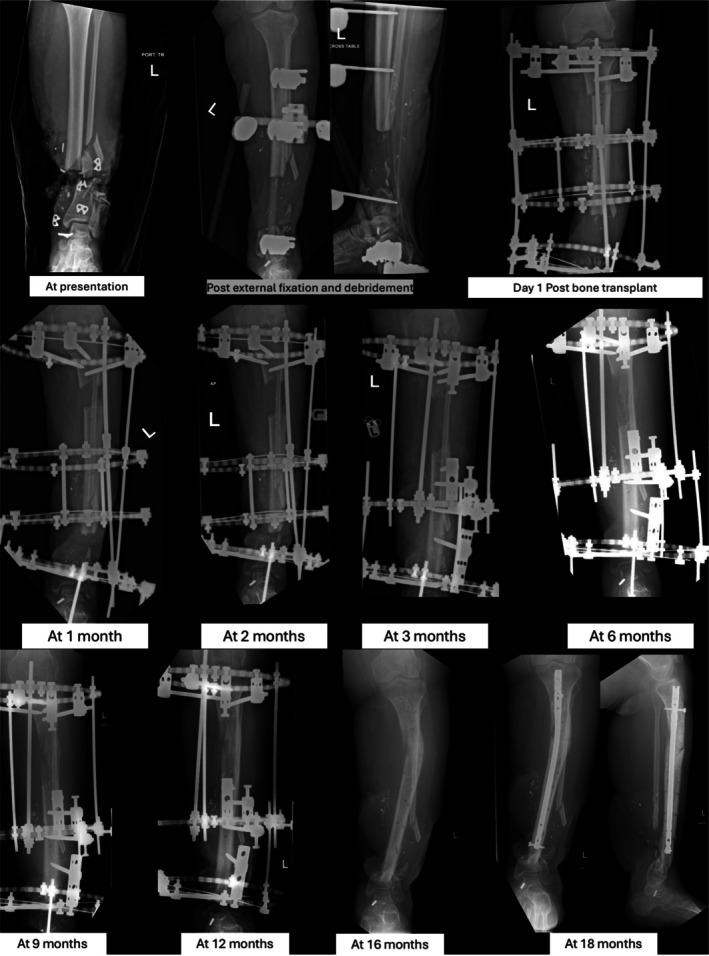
Serial Xray images showing bone transport process with Ilizraov frame.
